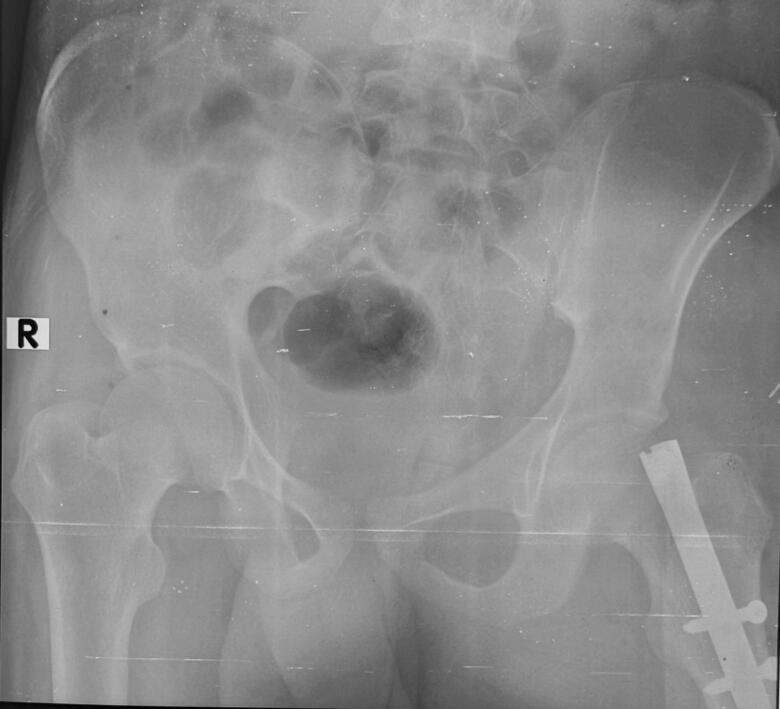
Post-operative x-ray pelvis with bilateral hip joint showing reduction of left hip.
